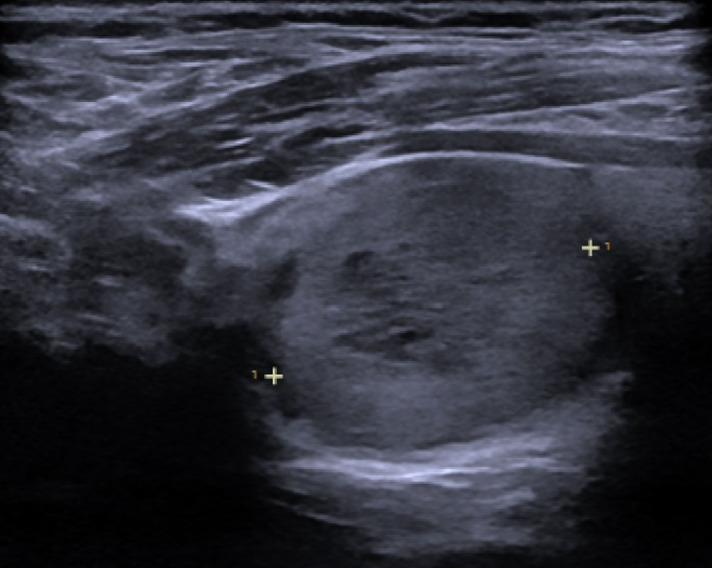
thyroid ultrasound: hypoechogenic, ovoid, solid thyroid nodule with well-defined margins
